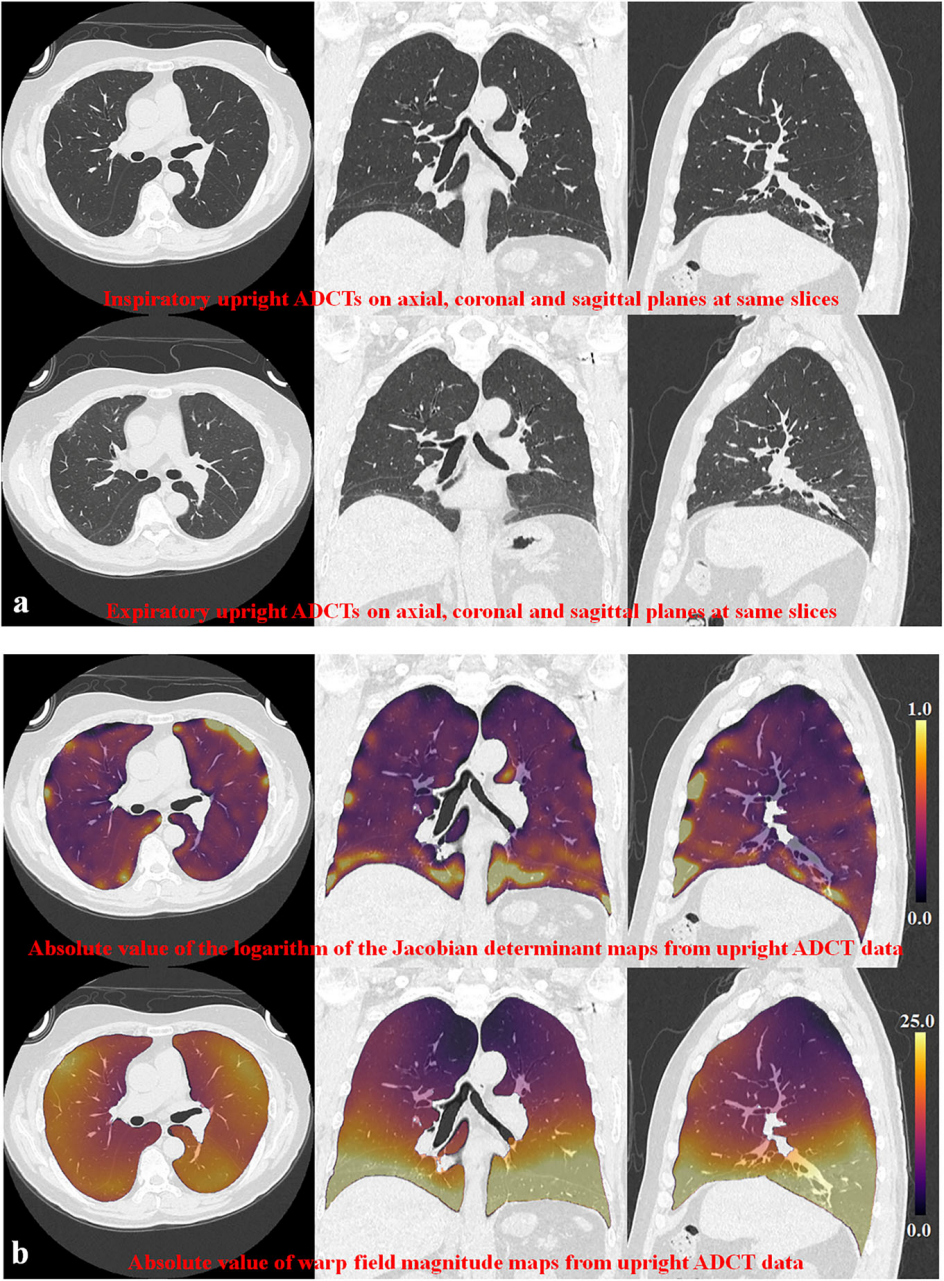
A 57-year-old female patient with polymyositis (%VC: 87%, %FVC: 89%, %FEV1: 81%, %DL_CO_: 84%, KL-6: 456U/mL, CT-assessed disease severity: 4). a 1st line: inspiratory upright ADCT; 2nd line: expiratory upright ADCT. Paired inspiratory-expiratory upright ADCT shows lung volume changes as well as lung shape, chest wall, and diaphragm positions. b 1st line: absolute value of the logarithm of the Jacobian determinant map; 2nd line: absolute value of the warp-field magnitude map. Both maps demonstrated larger absolute values of the logarithm of the Jacobian determinant and warp-field magnitude in the anterior than in the posterior lung areas, as well as the lower lung area being closer to the diaphragm than the upper lung area. *For acronyms, see the list of Abbreviations*.
